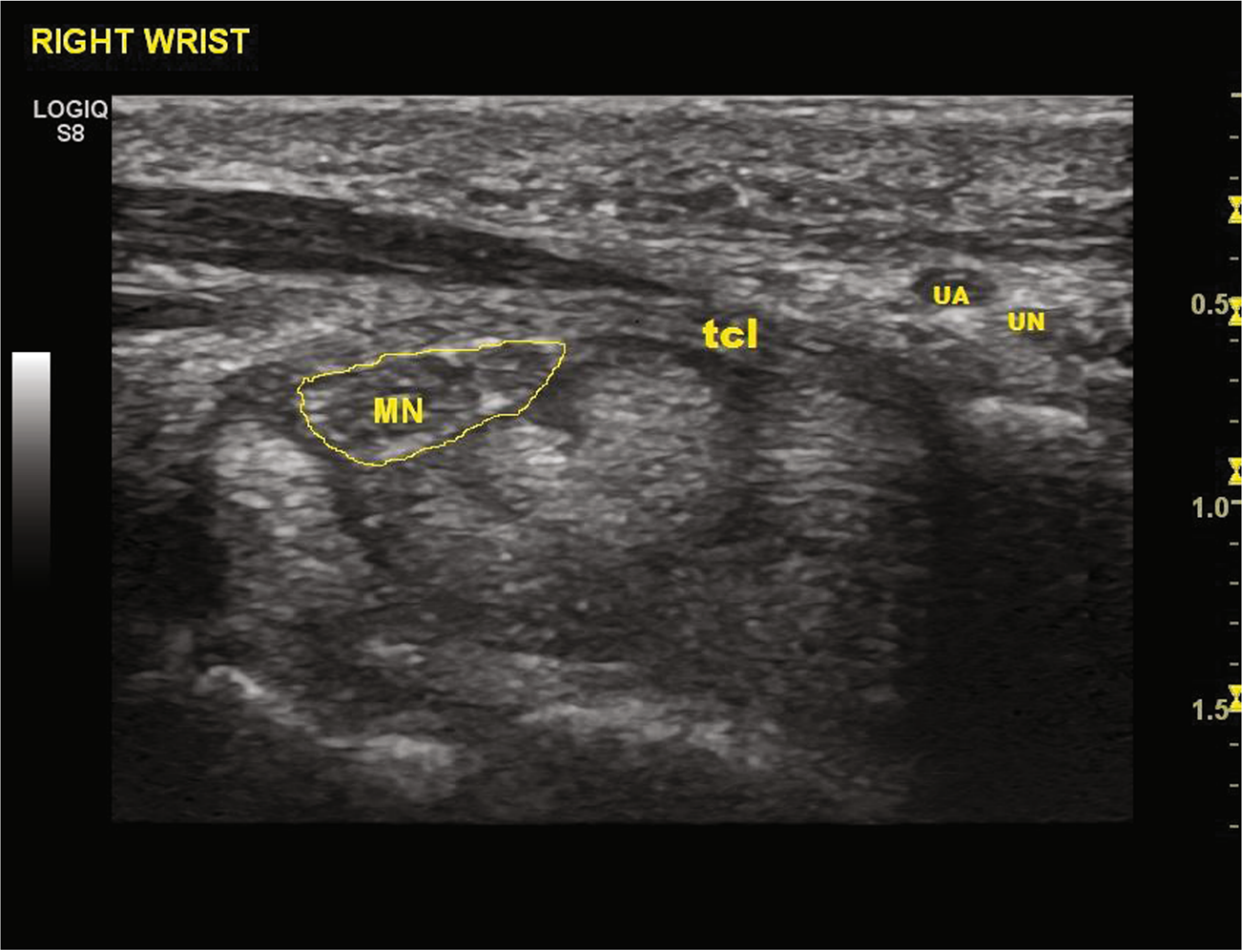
Ultrasound pre-examination of anatomy
